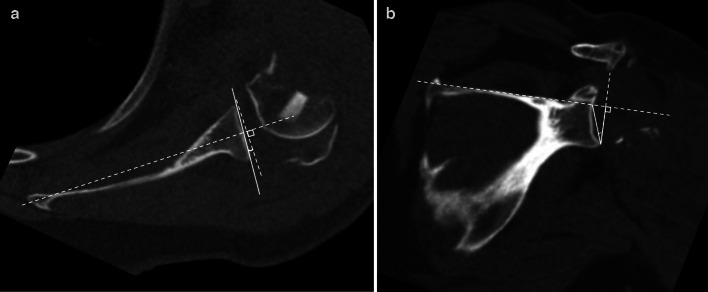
a Axial CT image demonstrating Glenoid Version measurement by the Friedman line technique. b Coronal CT image showing the reverse shoulder arthroplasty (RSA) angle of glenoid inclination by Boileau technique
